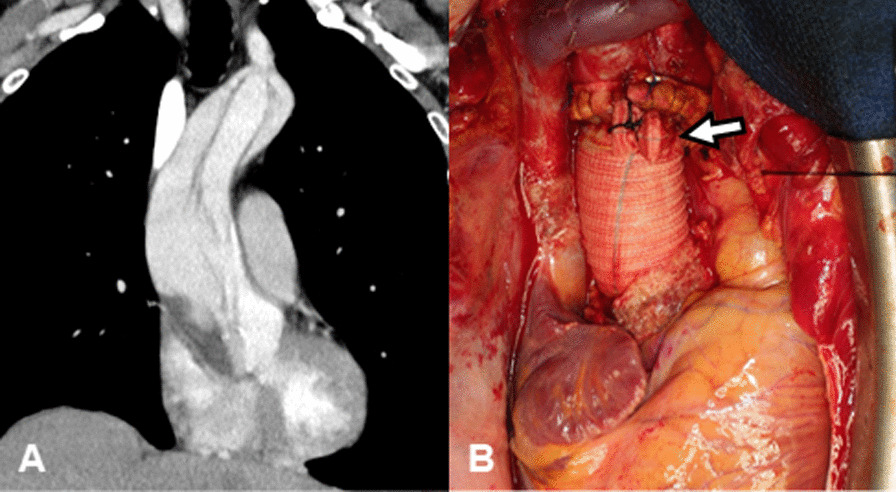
A Computed tomography angiography showing dissection of the ascending aorta. B Intraoperative photograph of the completed ascending aortic graft replacement. The side branch of the graft was ligated (arrow)
